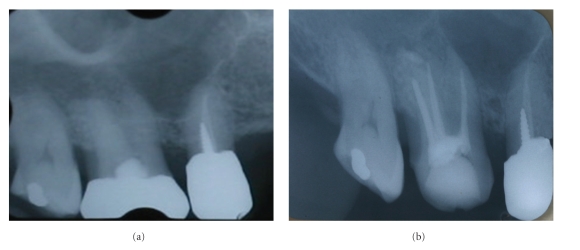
Radiography of tooth 16: (a) before treatment and (b) after root canal filling.
